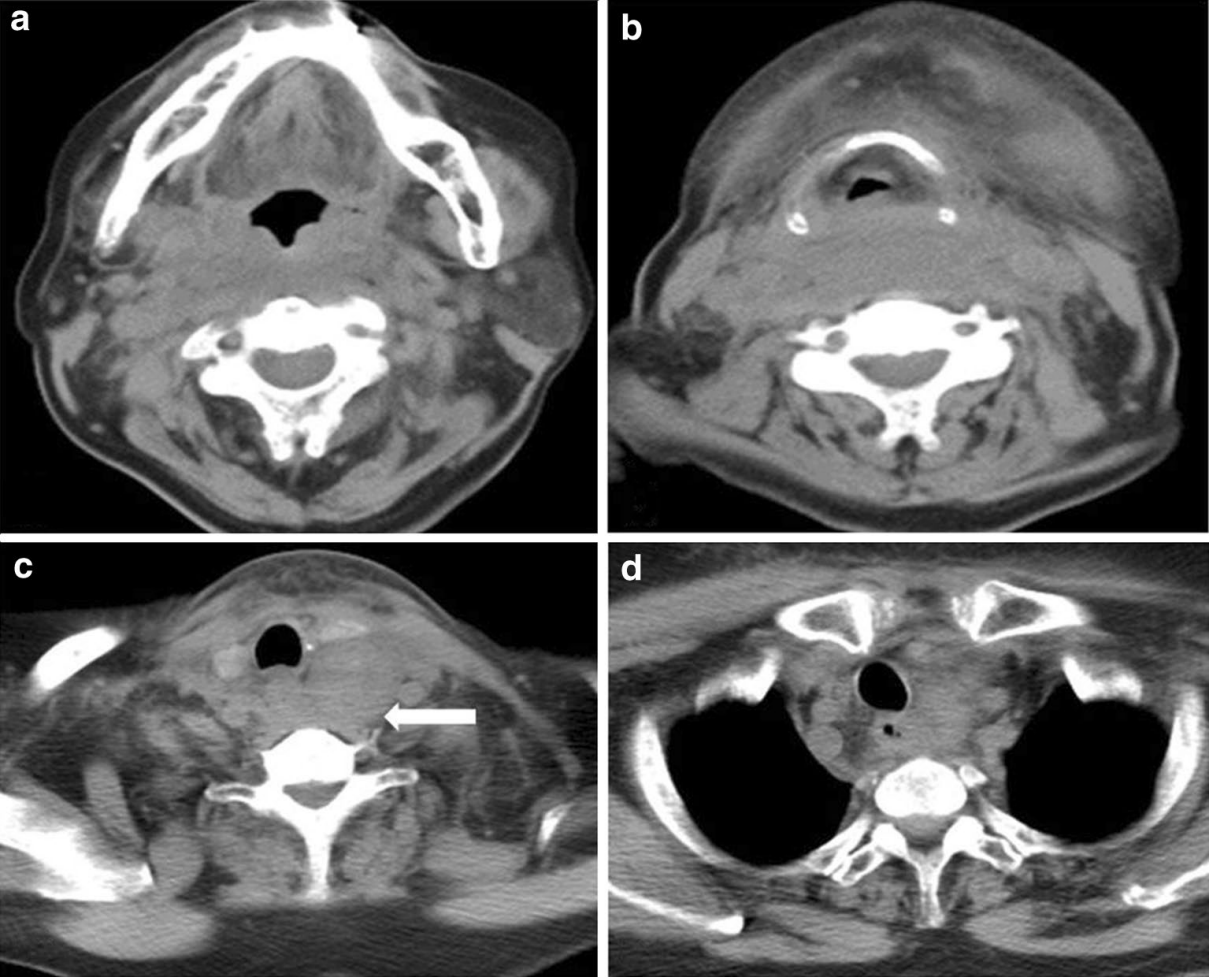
Case 1. Axial images of a CT of the neck and chest without contrast demonstrate diffuse soft tissue density posterior to the oropharynx, hypopharynx and larynx, displacing these structures anteriorly away from the spine (a, b). A more focal mass-like area of rounded soft tissue density is seen posterior to the left thyroid lobe, suggestive of a focal lesion (*arrow*) (c). Diffuse abnormal soft tissue density is also seen within the upper mediastinum (d)
